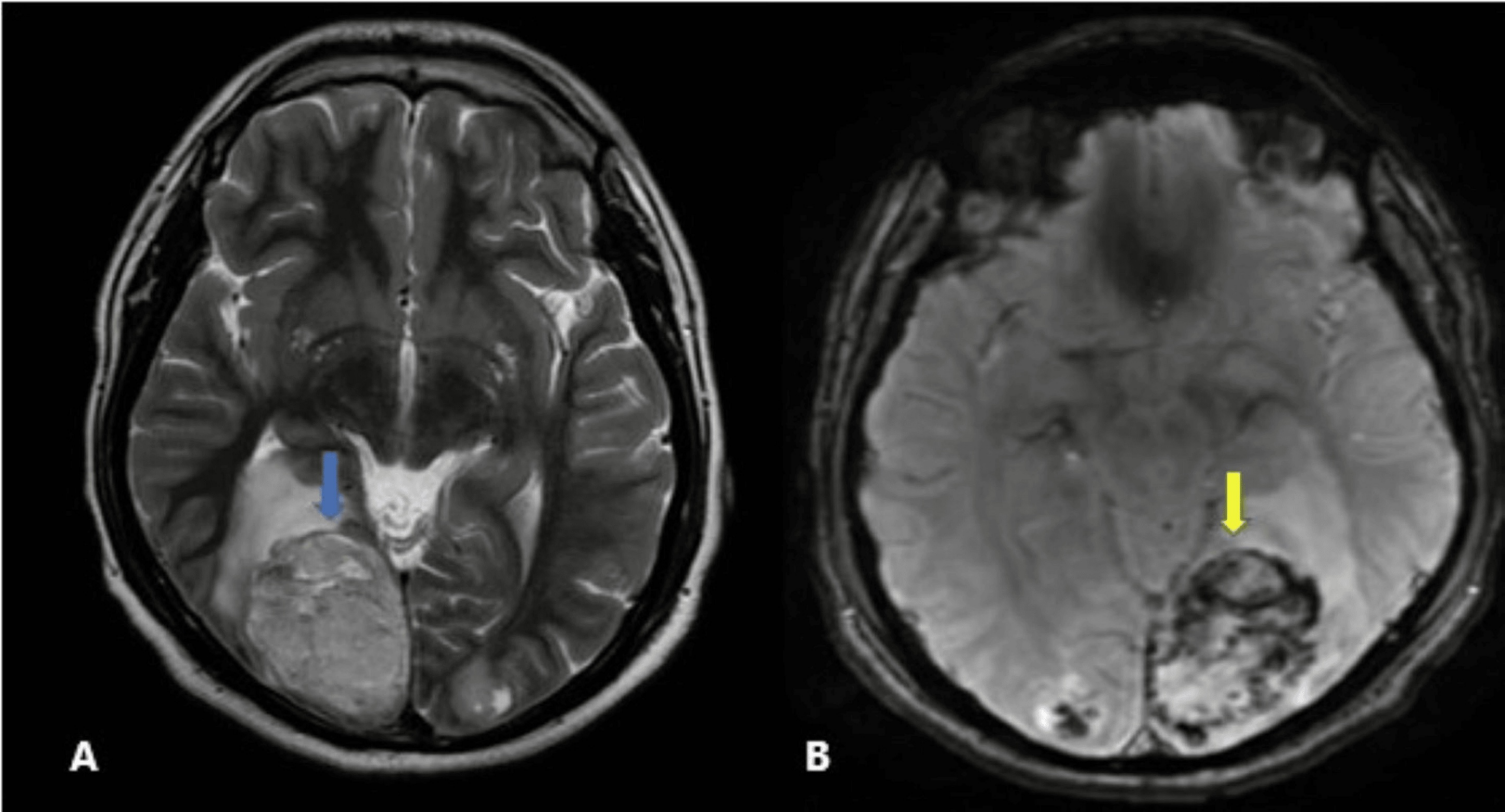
Radiological images (cranial MRI). (A) Axial T2 images showing a well-circumscribed heterogenous hyperintense lesion in the right posterior parietal lobe with surrounding edema (blue arrow). (B) GRE sequence demonstrating intralesional patchy hemorrhages (yellow arrow). MRI: magnetic resonance imaging, GRE: gradient echo
